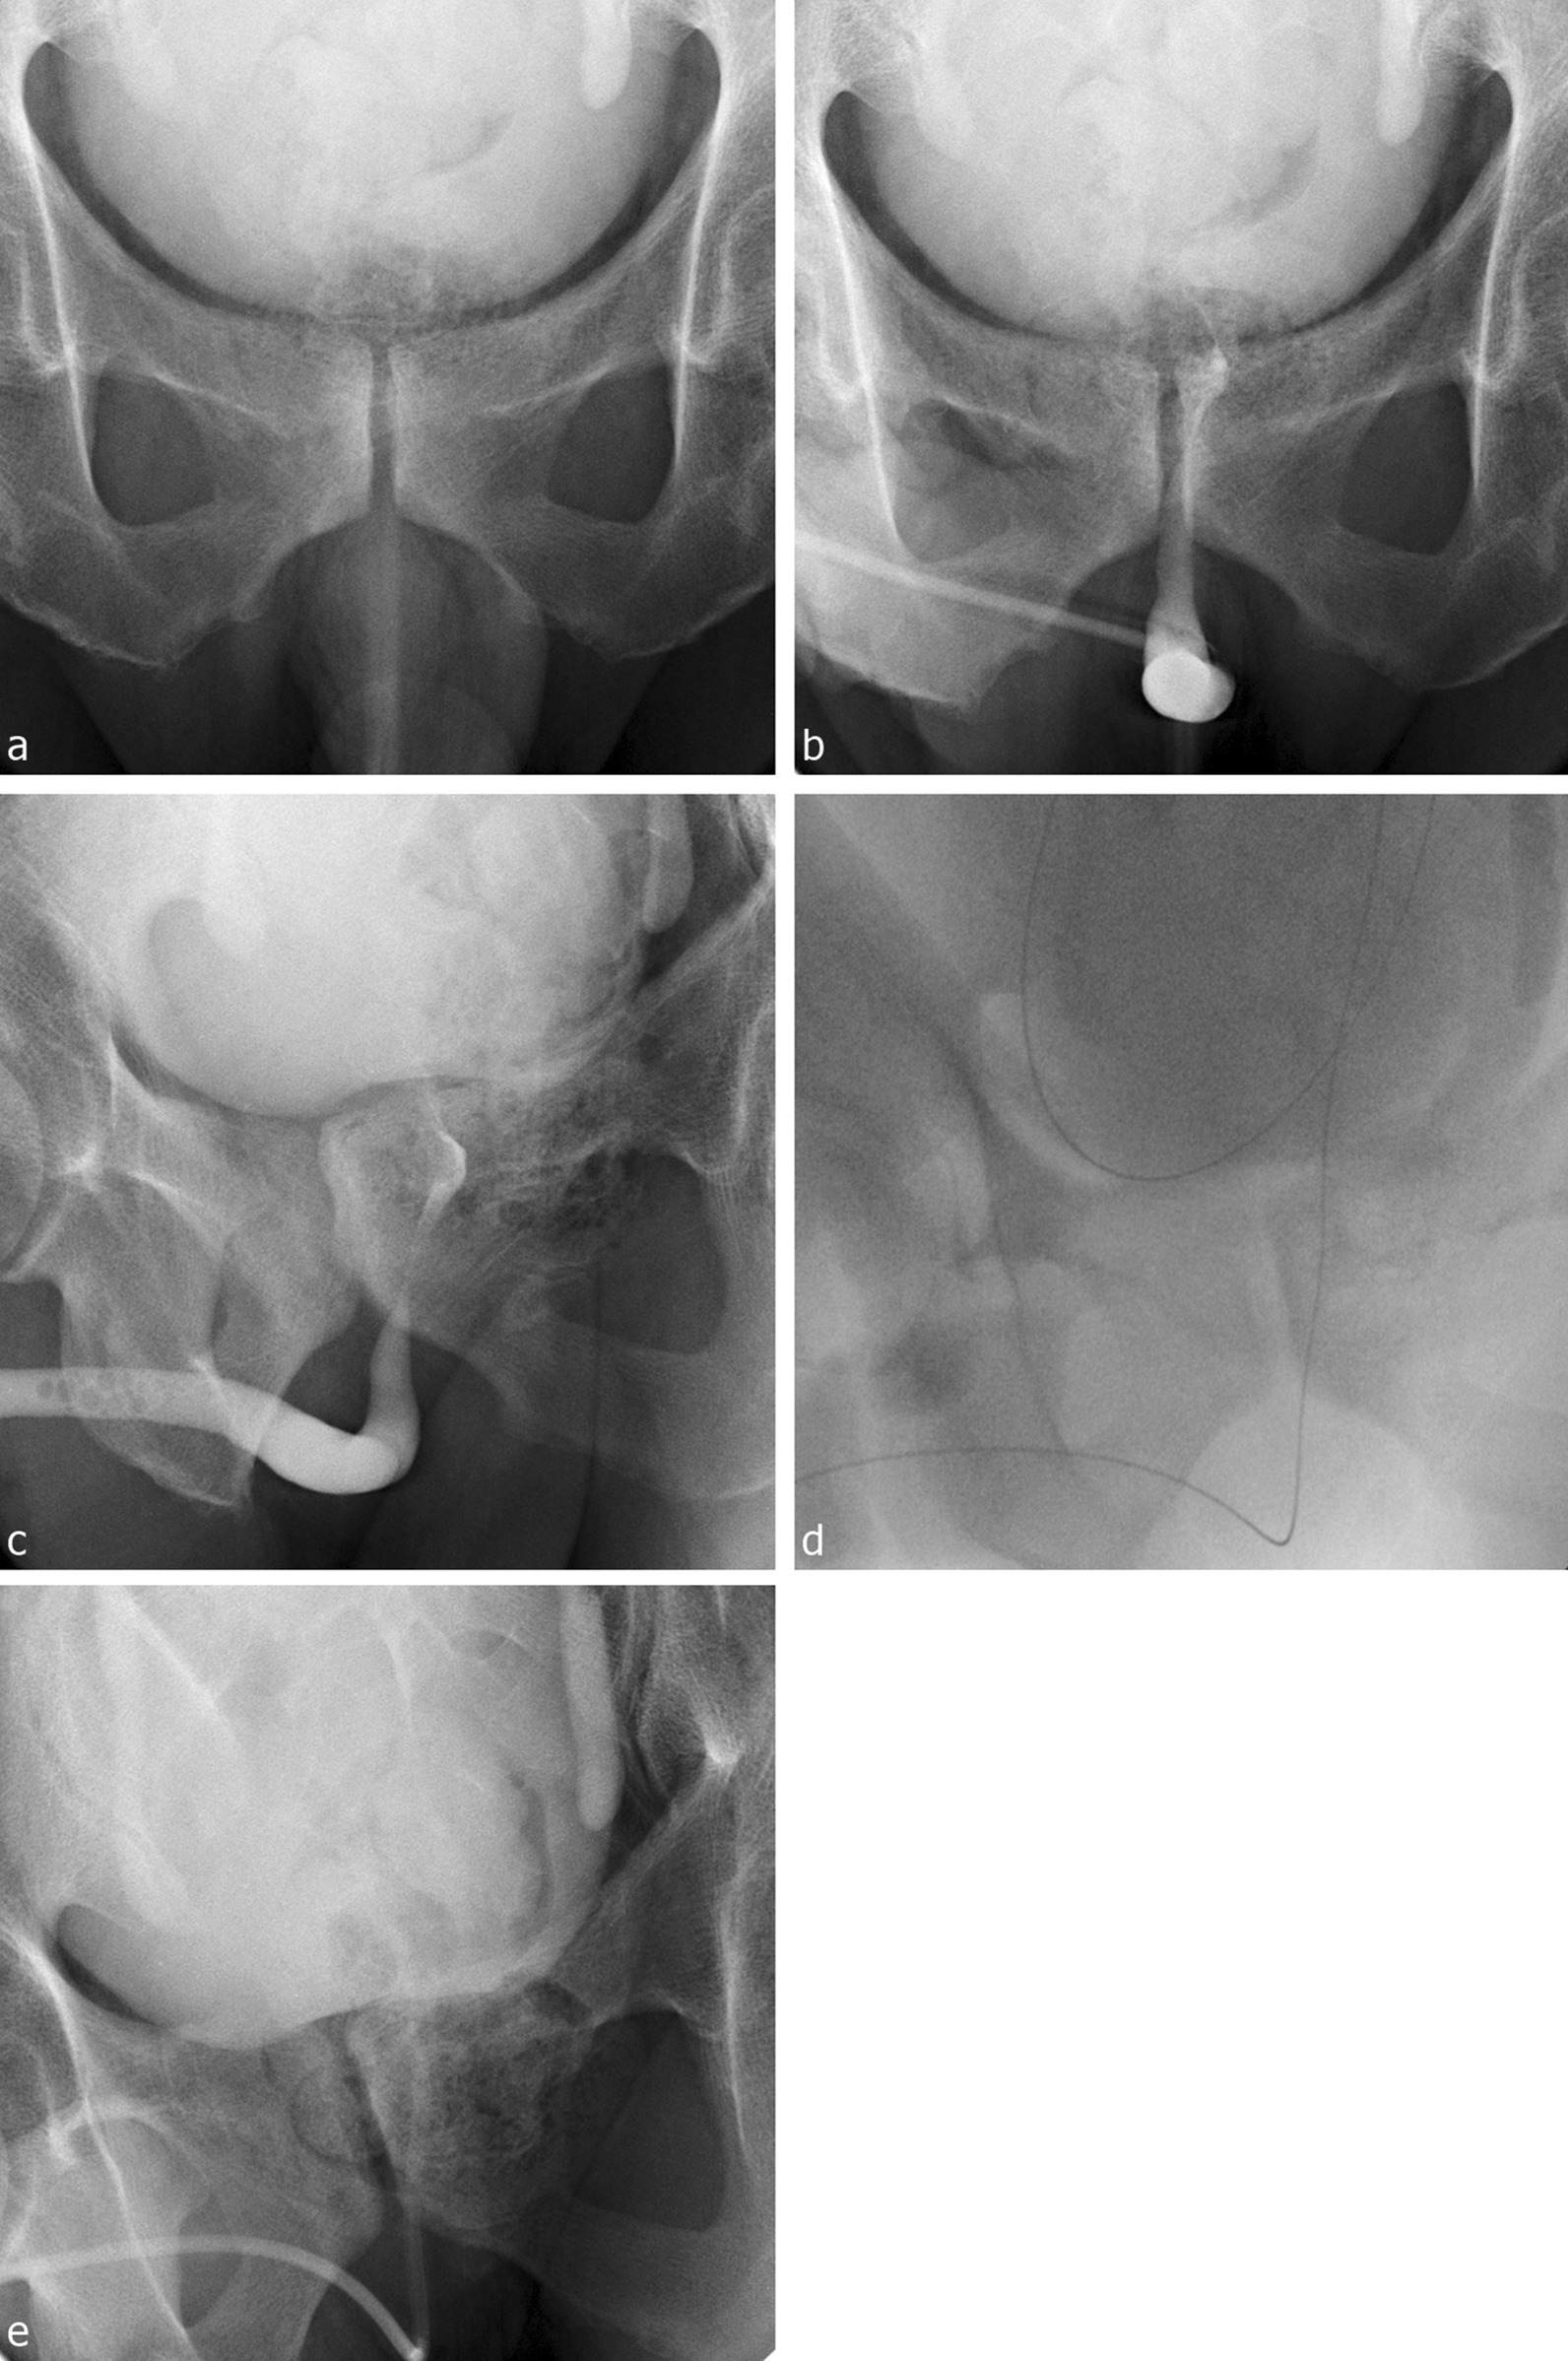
Urethral catheterization under RGU. a Contrast fluid remained in the bladder after enhanced computed tomography. b Narrowed prostatic urethra. c An oblique image of a narrowed prostatic urethra. c A hydrophilic guide wire was inserted into bladder under fluoroscopic guidance. d Foley catheter was inserted into the bladder using a hydrophilic guide wire
